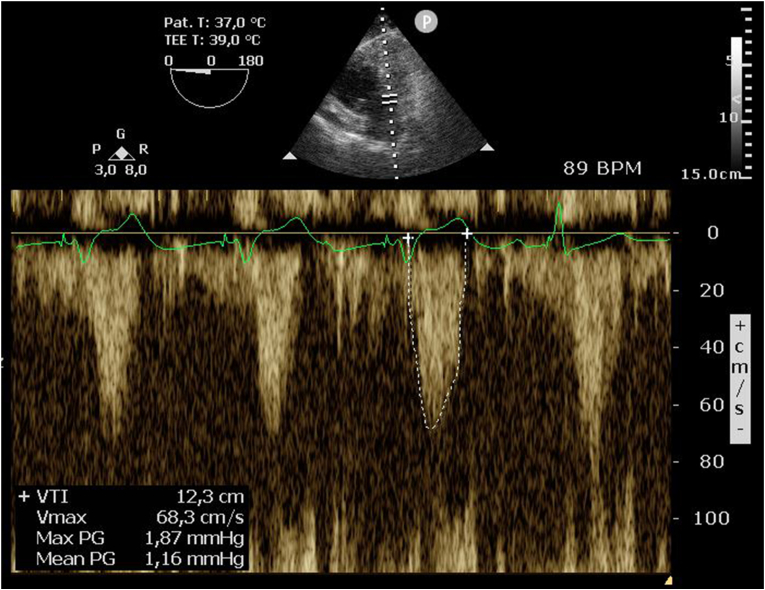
Perioperative measurement of surrogates of cardiac output with and without sRV pacing using transesophageal echocardiography. The RVOT VTI with and without sRV pacing was respectively 12.3 cm (at a heart rate of 89 bpm) and 14.7 cm (at 71 bpm). Max PG = peak pressure gradient; mean PG = mean pressure gradient; RVOT = right ventricular outflow tract; sRV = systemic right ventricle; Vmax = maximum velocity; VTI = velocity time integral.
